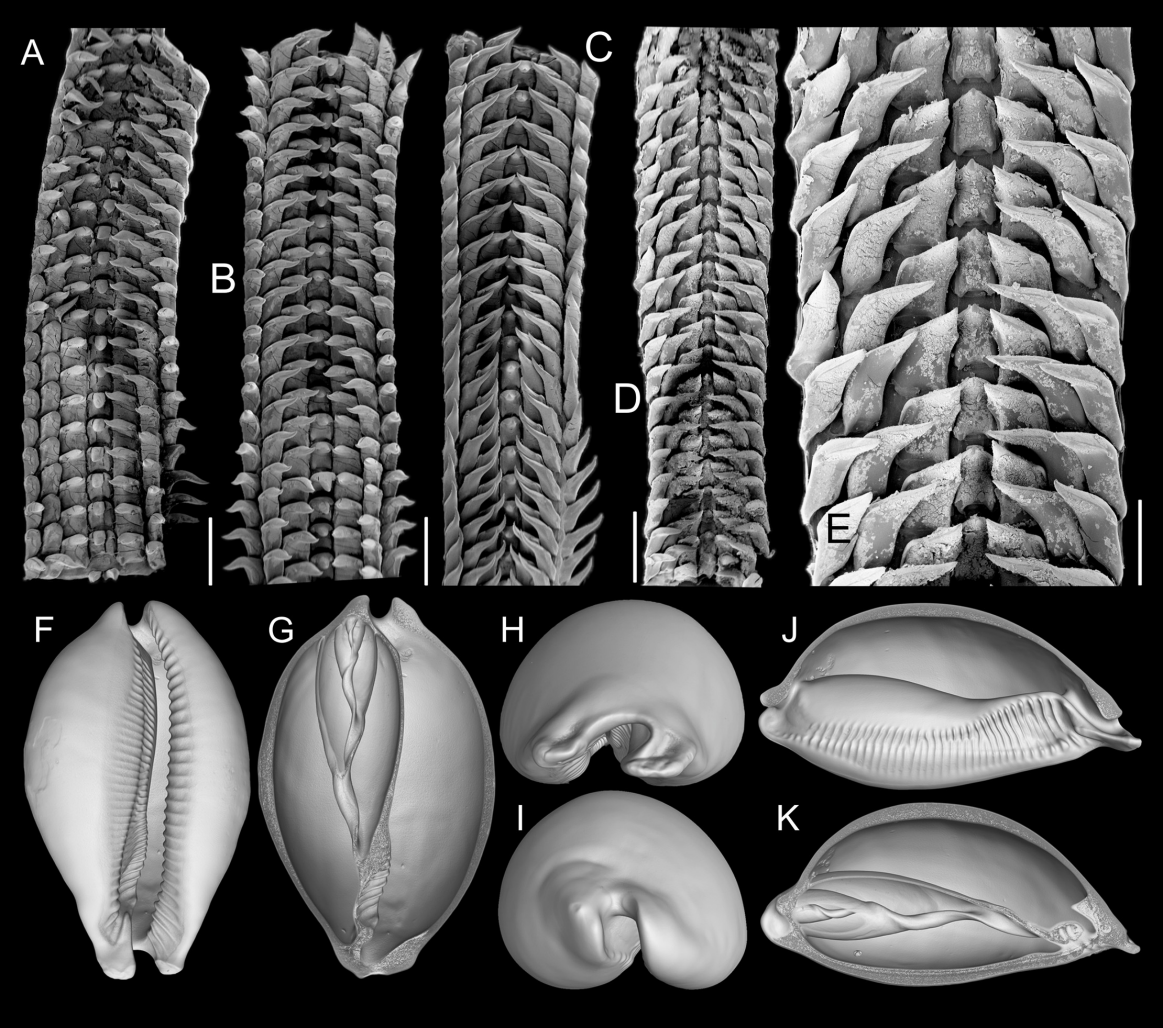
Macrocypraea* spp radulae in SEM. (A) *M*. *mammoth* paratype MZSP 137546; (B-C) *M*. *mammoth* holotype. (D) *M*. *cervus* ANSP 93276. (E) Same, detail. scales = 32–35: 1 mm, 36: 0.5 mm. (F-K) *M*. *mammoth* MZSP 137545, 3D reconstructions of shell (CT-Scan) (L 100.0 mm). |(F) Frontal view. (G) Same, more ventral portions removed. (H) Anterior view. (I) Posterior view. (J) Right view, right half removed. (K) Same, section along columellar axis.
